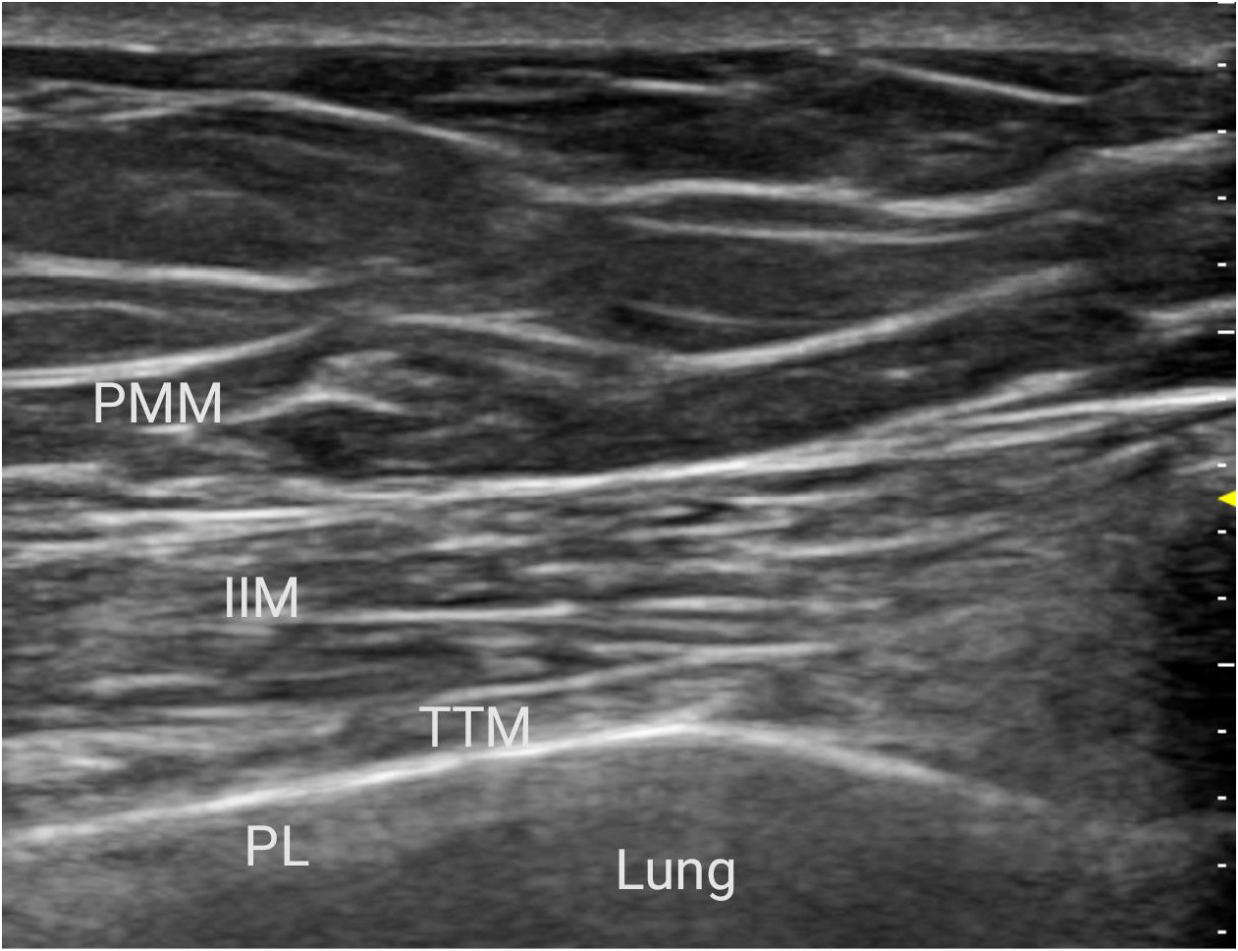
Ultrasound image of transversus thoracis muscle plane block. IIM, internal intercostal muscle; PL, pleura; PMM, pectoralis major muscle; TTM, transversus thoracis muscle.
